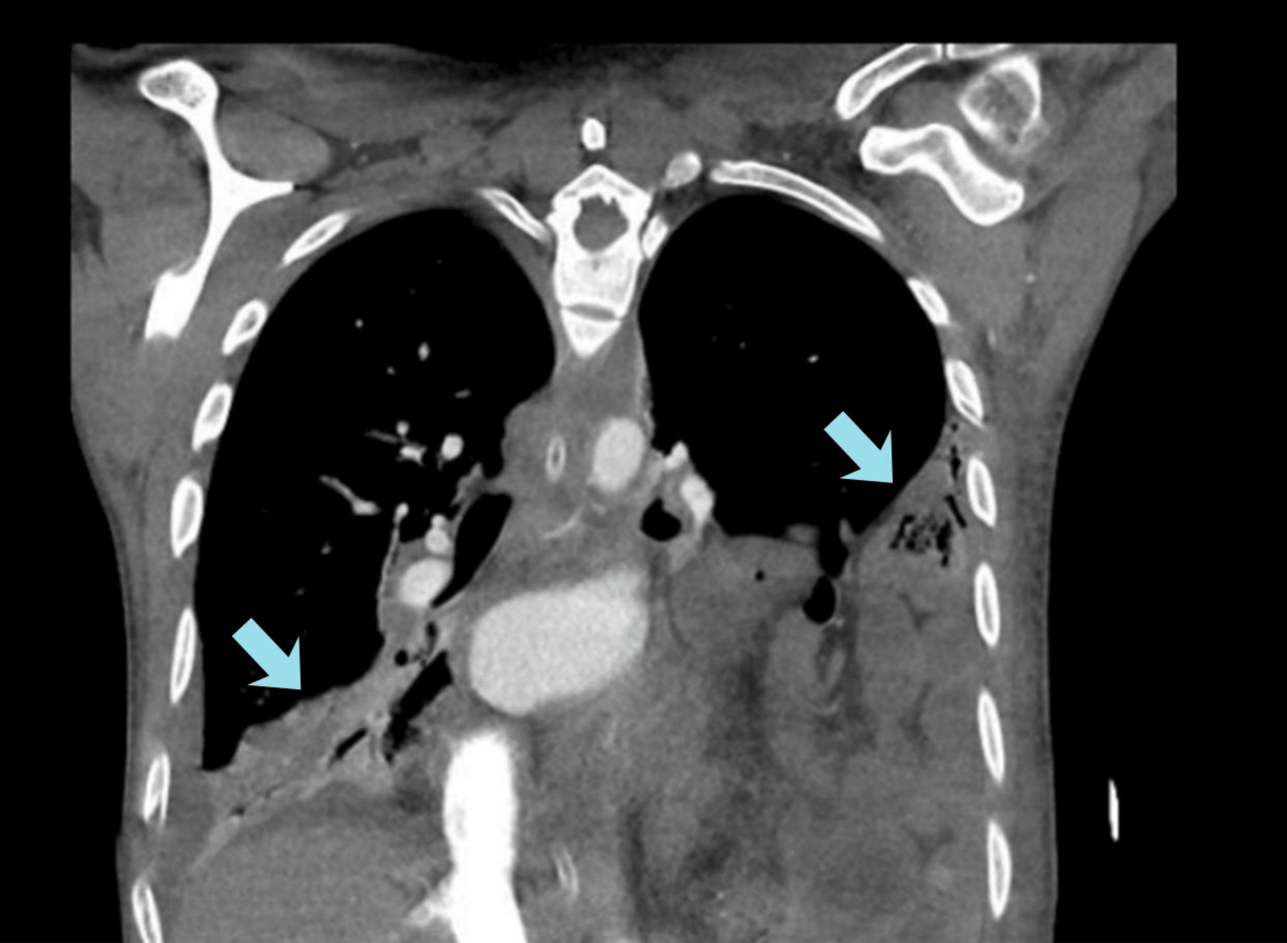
Computed tomography (CT) chest with intravenous contrast showing multiloculated high-density fluid collections formation, representing empyema, within the mediastinal region.
